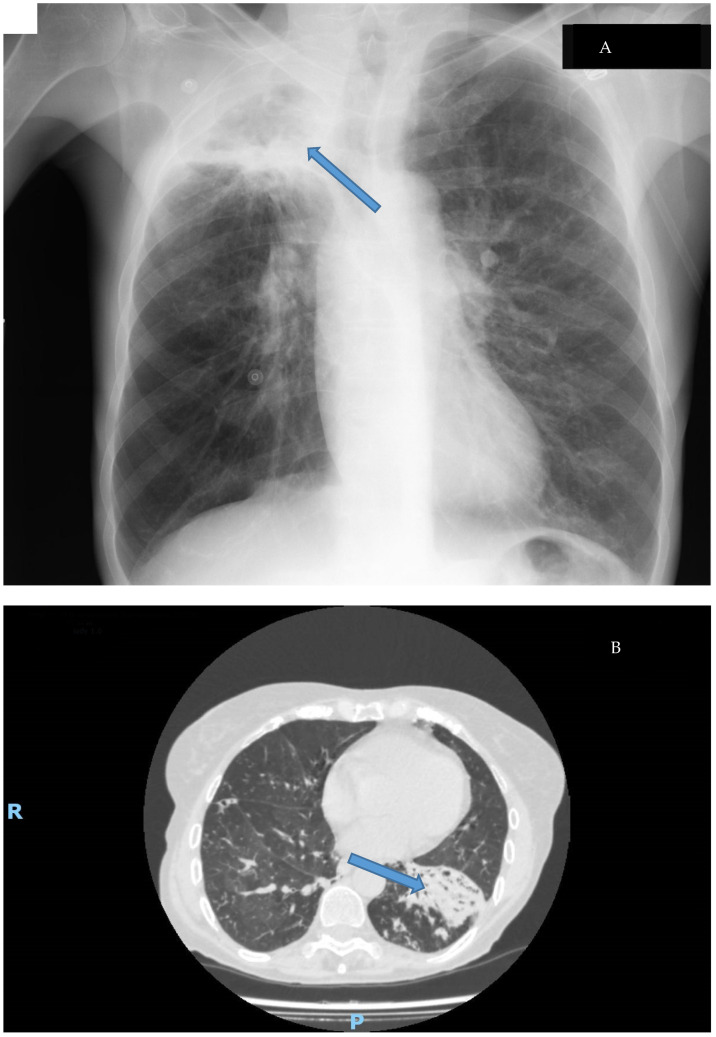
Types of lung disease caused by *M. abscessus*: (A): Cavitary disease (cavitation in the upper lobe of the right lung Thoracic X-ray); (B): Fibronodular disease (alveolar infiltrates with bronchiectasis in CT-Scan). Disease is signaled by an arrow.
